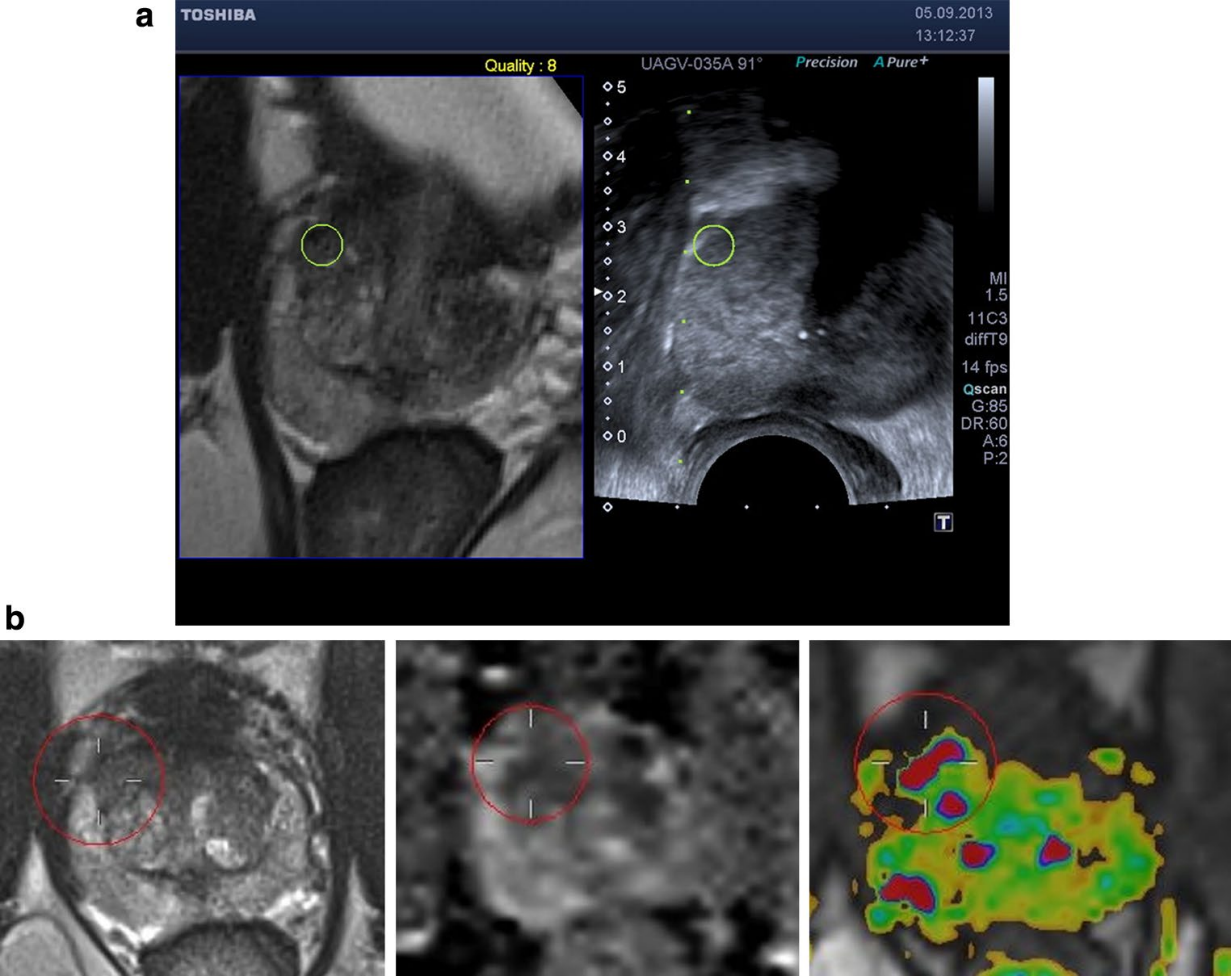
a Screenshot of the Toshiba Aplio 500 during MRgUSBx. The *green circle* indicates the target as reported on mpMRI, projected on the US image after fusion. The *dotted green line* indicates trajectory along which the needle will shoot in the prostate (to be moved slightly for correct targeting in this screenshot). b The corresponding mpMRI with from *left* to *right* the transversal T2-weighted image, ADC map, and DCE image. These images were displayed using ProCAD and were available during the fusion procedure
